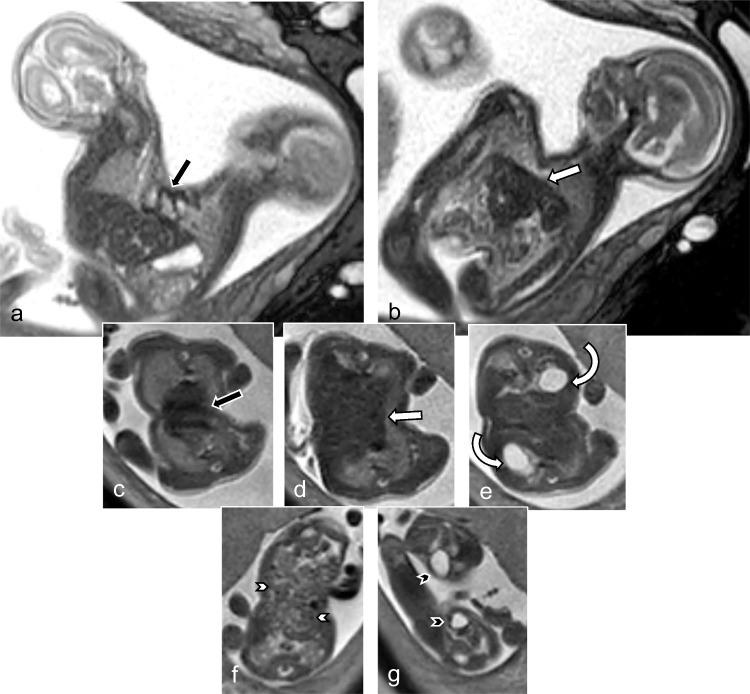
Fetal MRI of thoracopagus at gestational age 20 weeks, 5 days, showing ventral fusion of the chest and abdomen. a-b BTFE sagittal images of the ventrally conjoined chest and abdomen show a shared multi-chamber heart (*black arrow*) and shared liver (*white arrow*). c-f T2-weighted SSH TSE sequential axial images of the shared chest and abdomen show the shared heart (*black arrow*) with separate lungs, shared liver (*white arrow*), separate stomachs (*white curved arrows*), and shared peritoneal cavity with bowel (*white arrowheads*). g T2-weighted SSH TSE axial image through separate pelvises shows separate urinary bladders (*black arrowheads*). *BTFE*, balanced turbo-field echo; *SSH TSE*, single-shot turbo spin-echo
